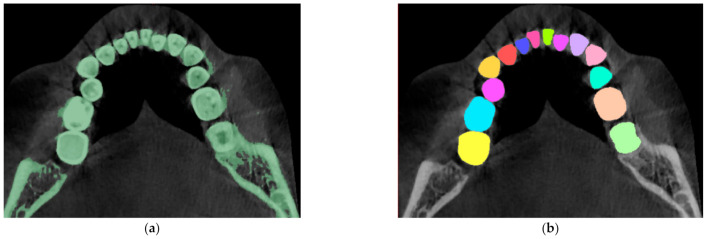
The result of one segmentation of the computed tomography (CT) scans: (a) automatic segmentation using the 1000–3000 Hounsfield units (HU) range; (b) the result of interactive image editing (selection of areas related to the teeth of the mandible, on the right).
